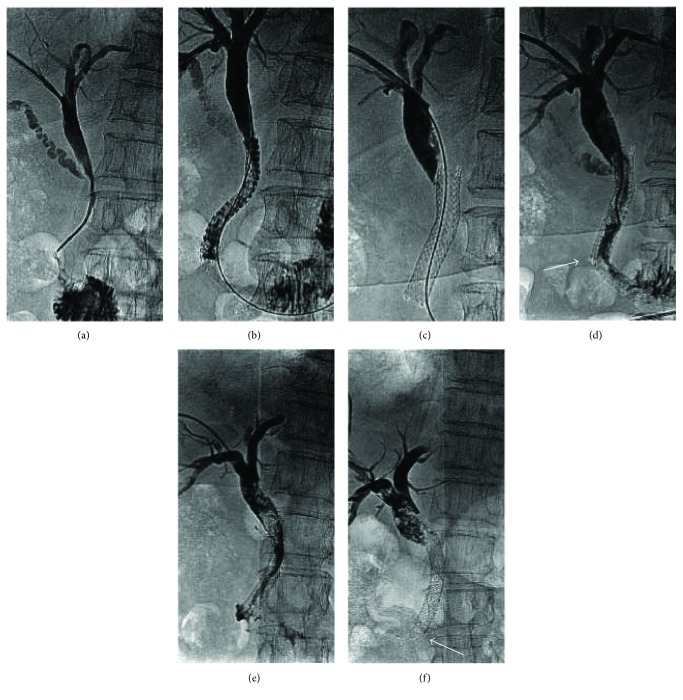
Complete stent fracture in an 81-year-old woman with common bile duct obstruction secondary to a cystic mass of the pancreatic head. (a). Percutaneous transhepatic cholangiogram depicting common bile duct stricture was shown. (b). Six weeks later, predilation (diameter, 8 mm; length, 40 mm; ATB5-35-40-8-4.0; COOK Inc.) was performed and a stent (diameter, 8 mm; length, 60 mm; COOK Inc., Bloomington, IN, United States) was inserted in the stricture, extending from the duodenum to the cystic duct. (c, d). Eleven months after biliary stent placement, the patient developed reobstructive jaundice, and percutaneous cholangiography showed stent occlusion. Two additional stents (diameter, 8 mm; length, 60 mm; COOK Inc., Bloomington, IN, United States) were deployed. An obtuse angle was formed distally between the two biliary stents (arrow). (e, f). Thirty-seven days after implantation of the two biliary stents, plain X-ray revealed the absence of the distal part of the stent (arrow), resulting from complete stent fracture. The fractured stent had therefore passed through the bowel without incident.
